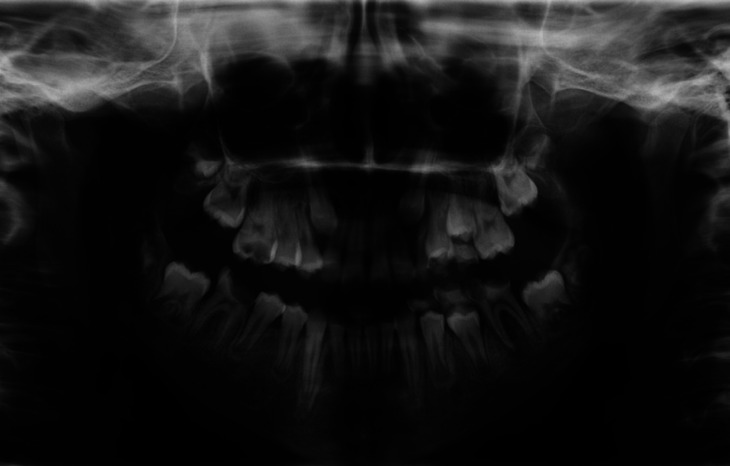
Orthopantomography showing mixed dentition and multiple dental caries in both primary and permanent dentition; periodontal diseases is also visible, along with crowding in the upper jaw leading to malocclusion.
